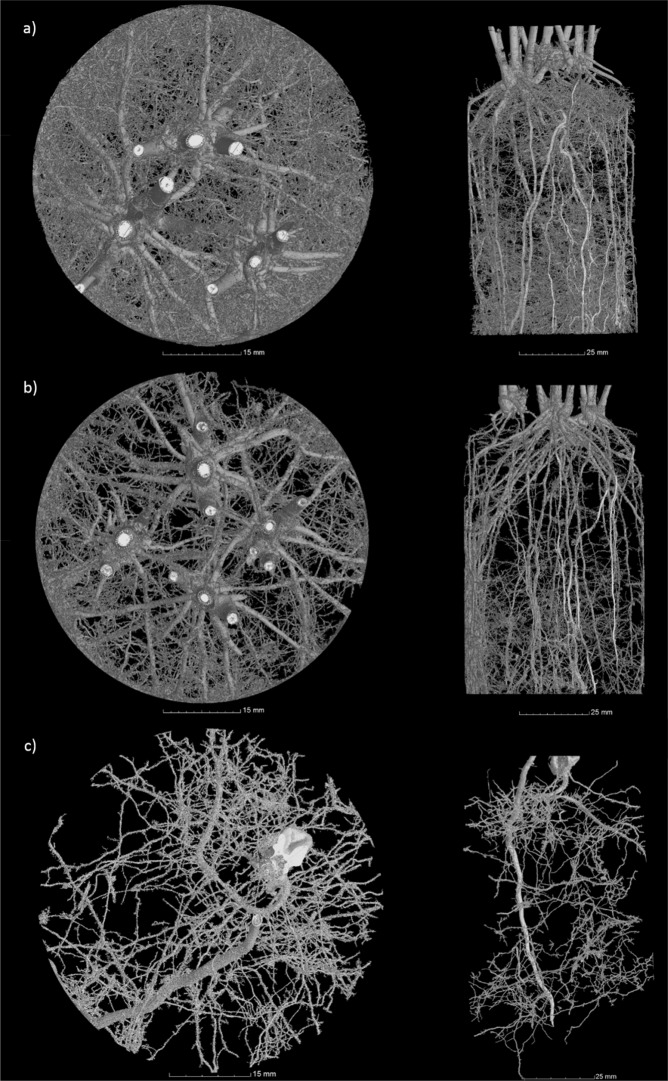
Example xy and zx view images from X-ray Computed Tomography scans of Palisade grass (a), Ruzigrass (b) and Maize (c).
